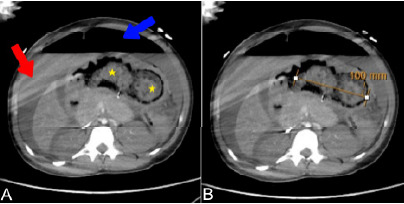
Abdominal computed tomography (axial plane) images at the level of the gastric outlet. (A) Free fluid (red arrow), pneumoperitoneum (blue arrow), and a large intragastric mass (yellow stars) are visible. (B) Radiological measurement of the intragastric mass demonstrates a diameter of approximately 100 millimeters.
